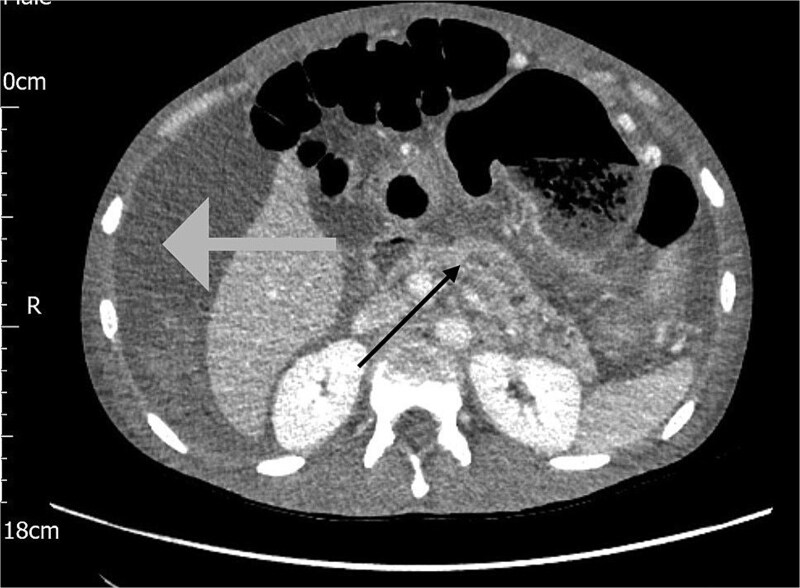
CT scan of the abdomen showing haemorrhagic ascites in the perihepatic area (grey arrow) and an irregular dilated pancreatic duct (black arrow).
